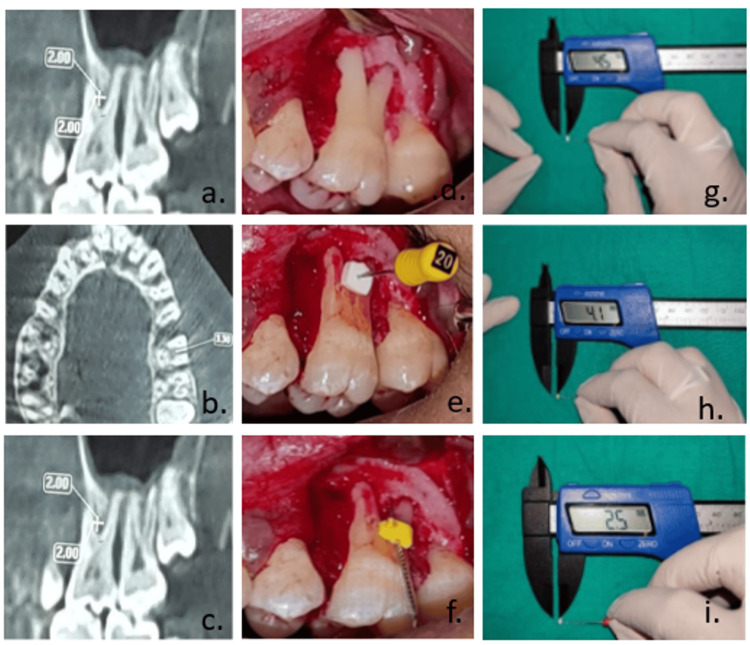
Measurement process using CBCT and Vernier caliper. (a-c) CBCT measurements of width, depth, and height, respectively. (d-i) Intrasurgical measurements transferred to Vernier caliper for precise assessment of width, depth, and height CBCT: cone-beam computed tomography
